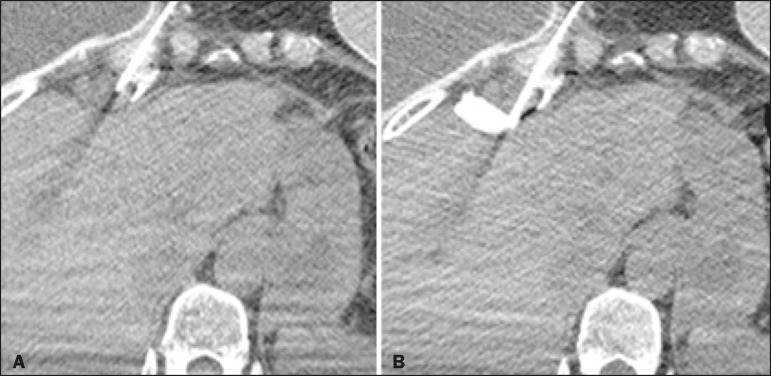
CT-guided percutaneous biopsy of a lymph node in the internal thoracic chain. A: Pre-procedure planning. B: Hydrodissection with lidocaine gel and iodinated contrast.
